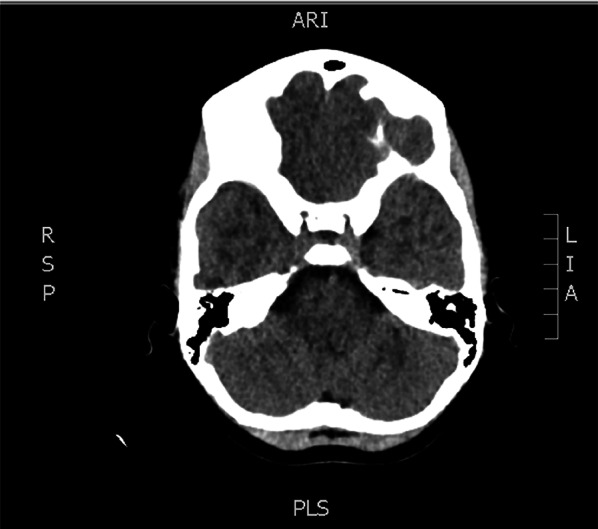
Patients CT scan showing normal cerebral parenchyma with no evidence of haemorrhage, space-occupying lesion, infarct, or intra or extra-axial fluid collections
